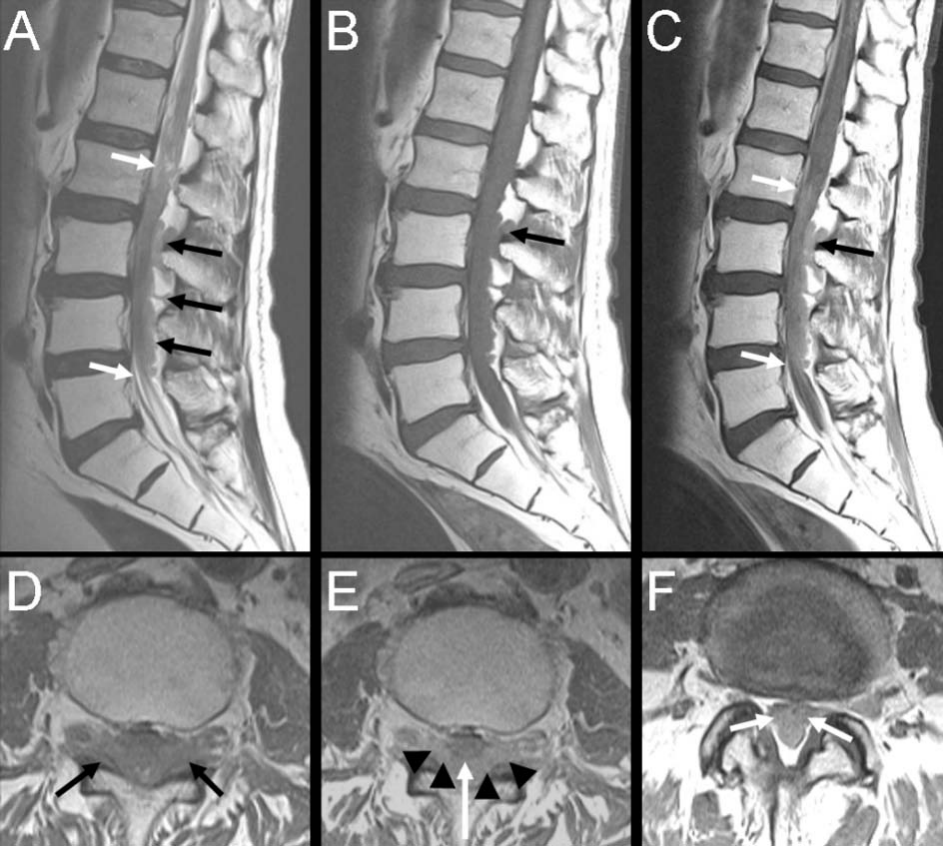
Magnetic resonance imaging (MRI) scan of the lumbar spine eight months after the onset of symptoms. T2-weighted (A) and T1-weighted (B-C) images before and after contrast administration show mild contrast enhancing multilobular tumors in the epidural fat behind the dural sac at the level of L3-L4 (black arrows) and a dural sac filled with intradural tumors (white arrows). Note the absence of the normal cerebrospinal fluid signal in the dural sac below the medullary conus. Axial T1-weighted images before (D) and after (E) contrast administration show the epidural metastases lateral and dorsal to the dural sac (black arrows in D; arrowheads in E). The white arrow in E shows the dorsal limit of the dural sac. F) Axial T1-weighted image after contrast administration shows a tumor-filled dural sac (white arrows).
